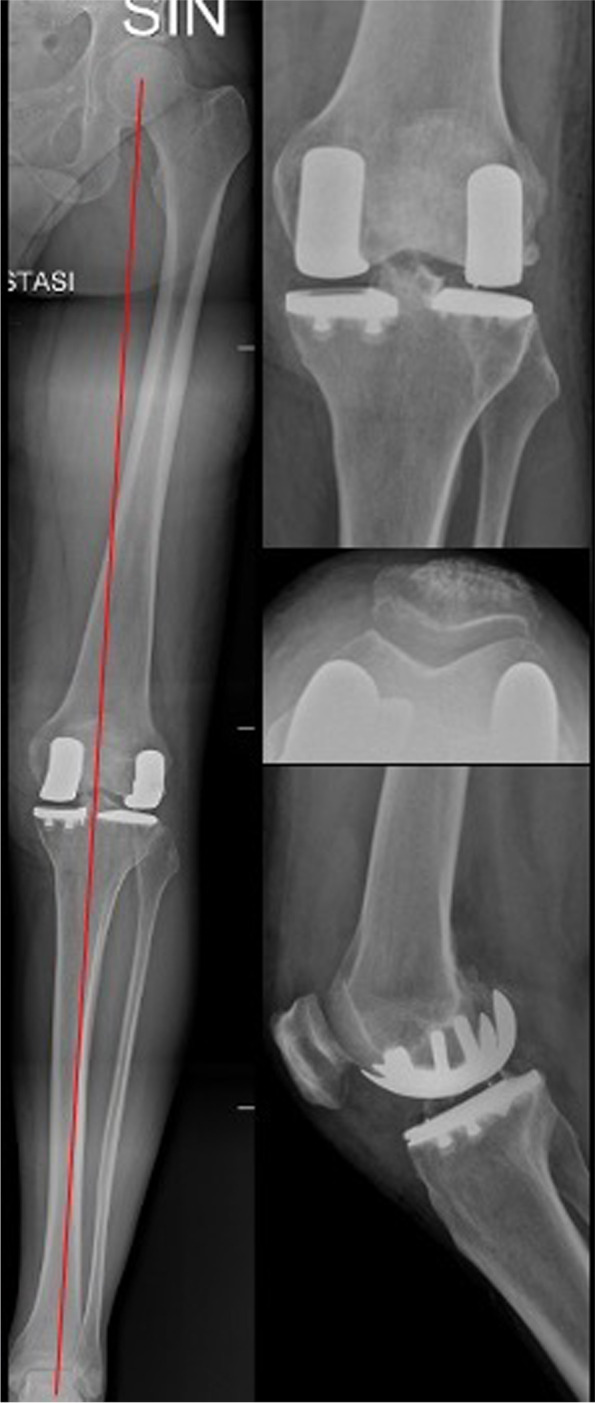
Postoperative X-rays evaluation of the case in Fig. 1. It is possible to see the respect of the native morphotype and the different prosthetic components used in the medial and in the lateral compartments
